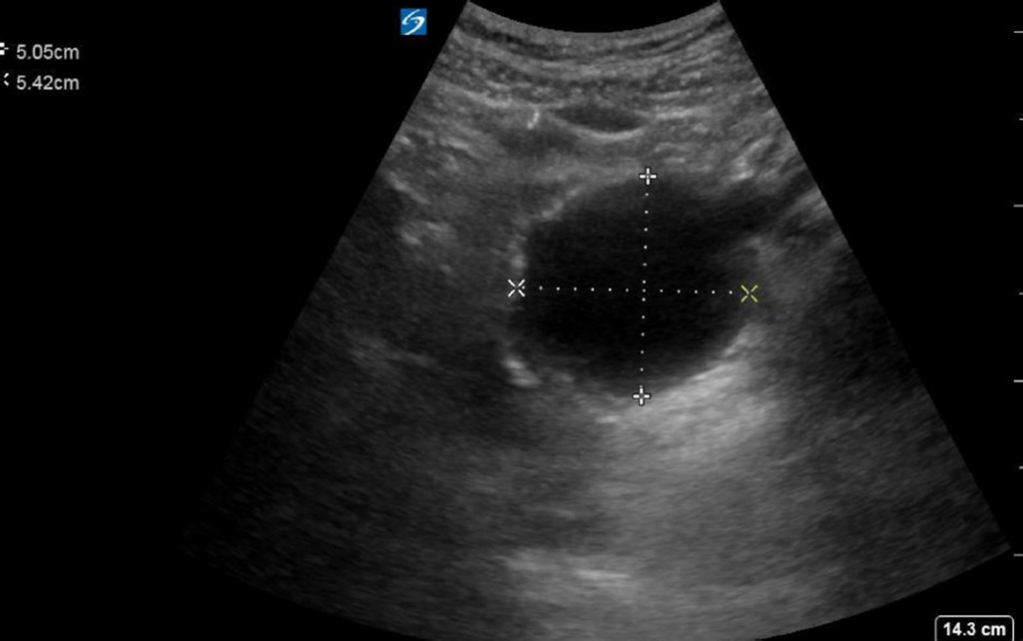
Point of care ultrasound (POCUS) demonstrating diameter measurements for an ovarian cyst (5.05 x 5.42 cm).
